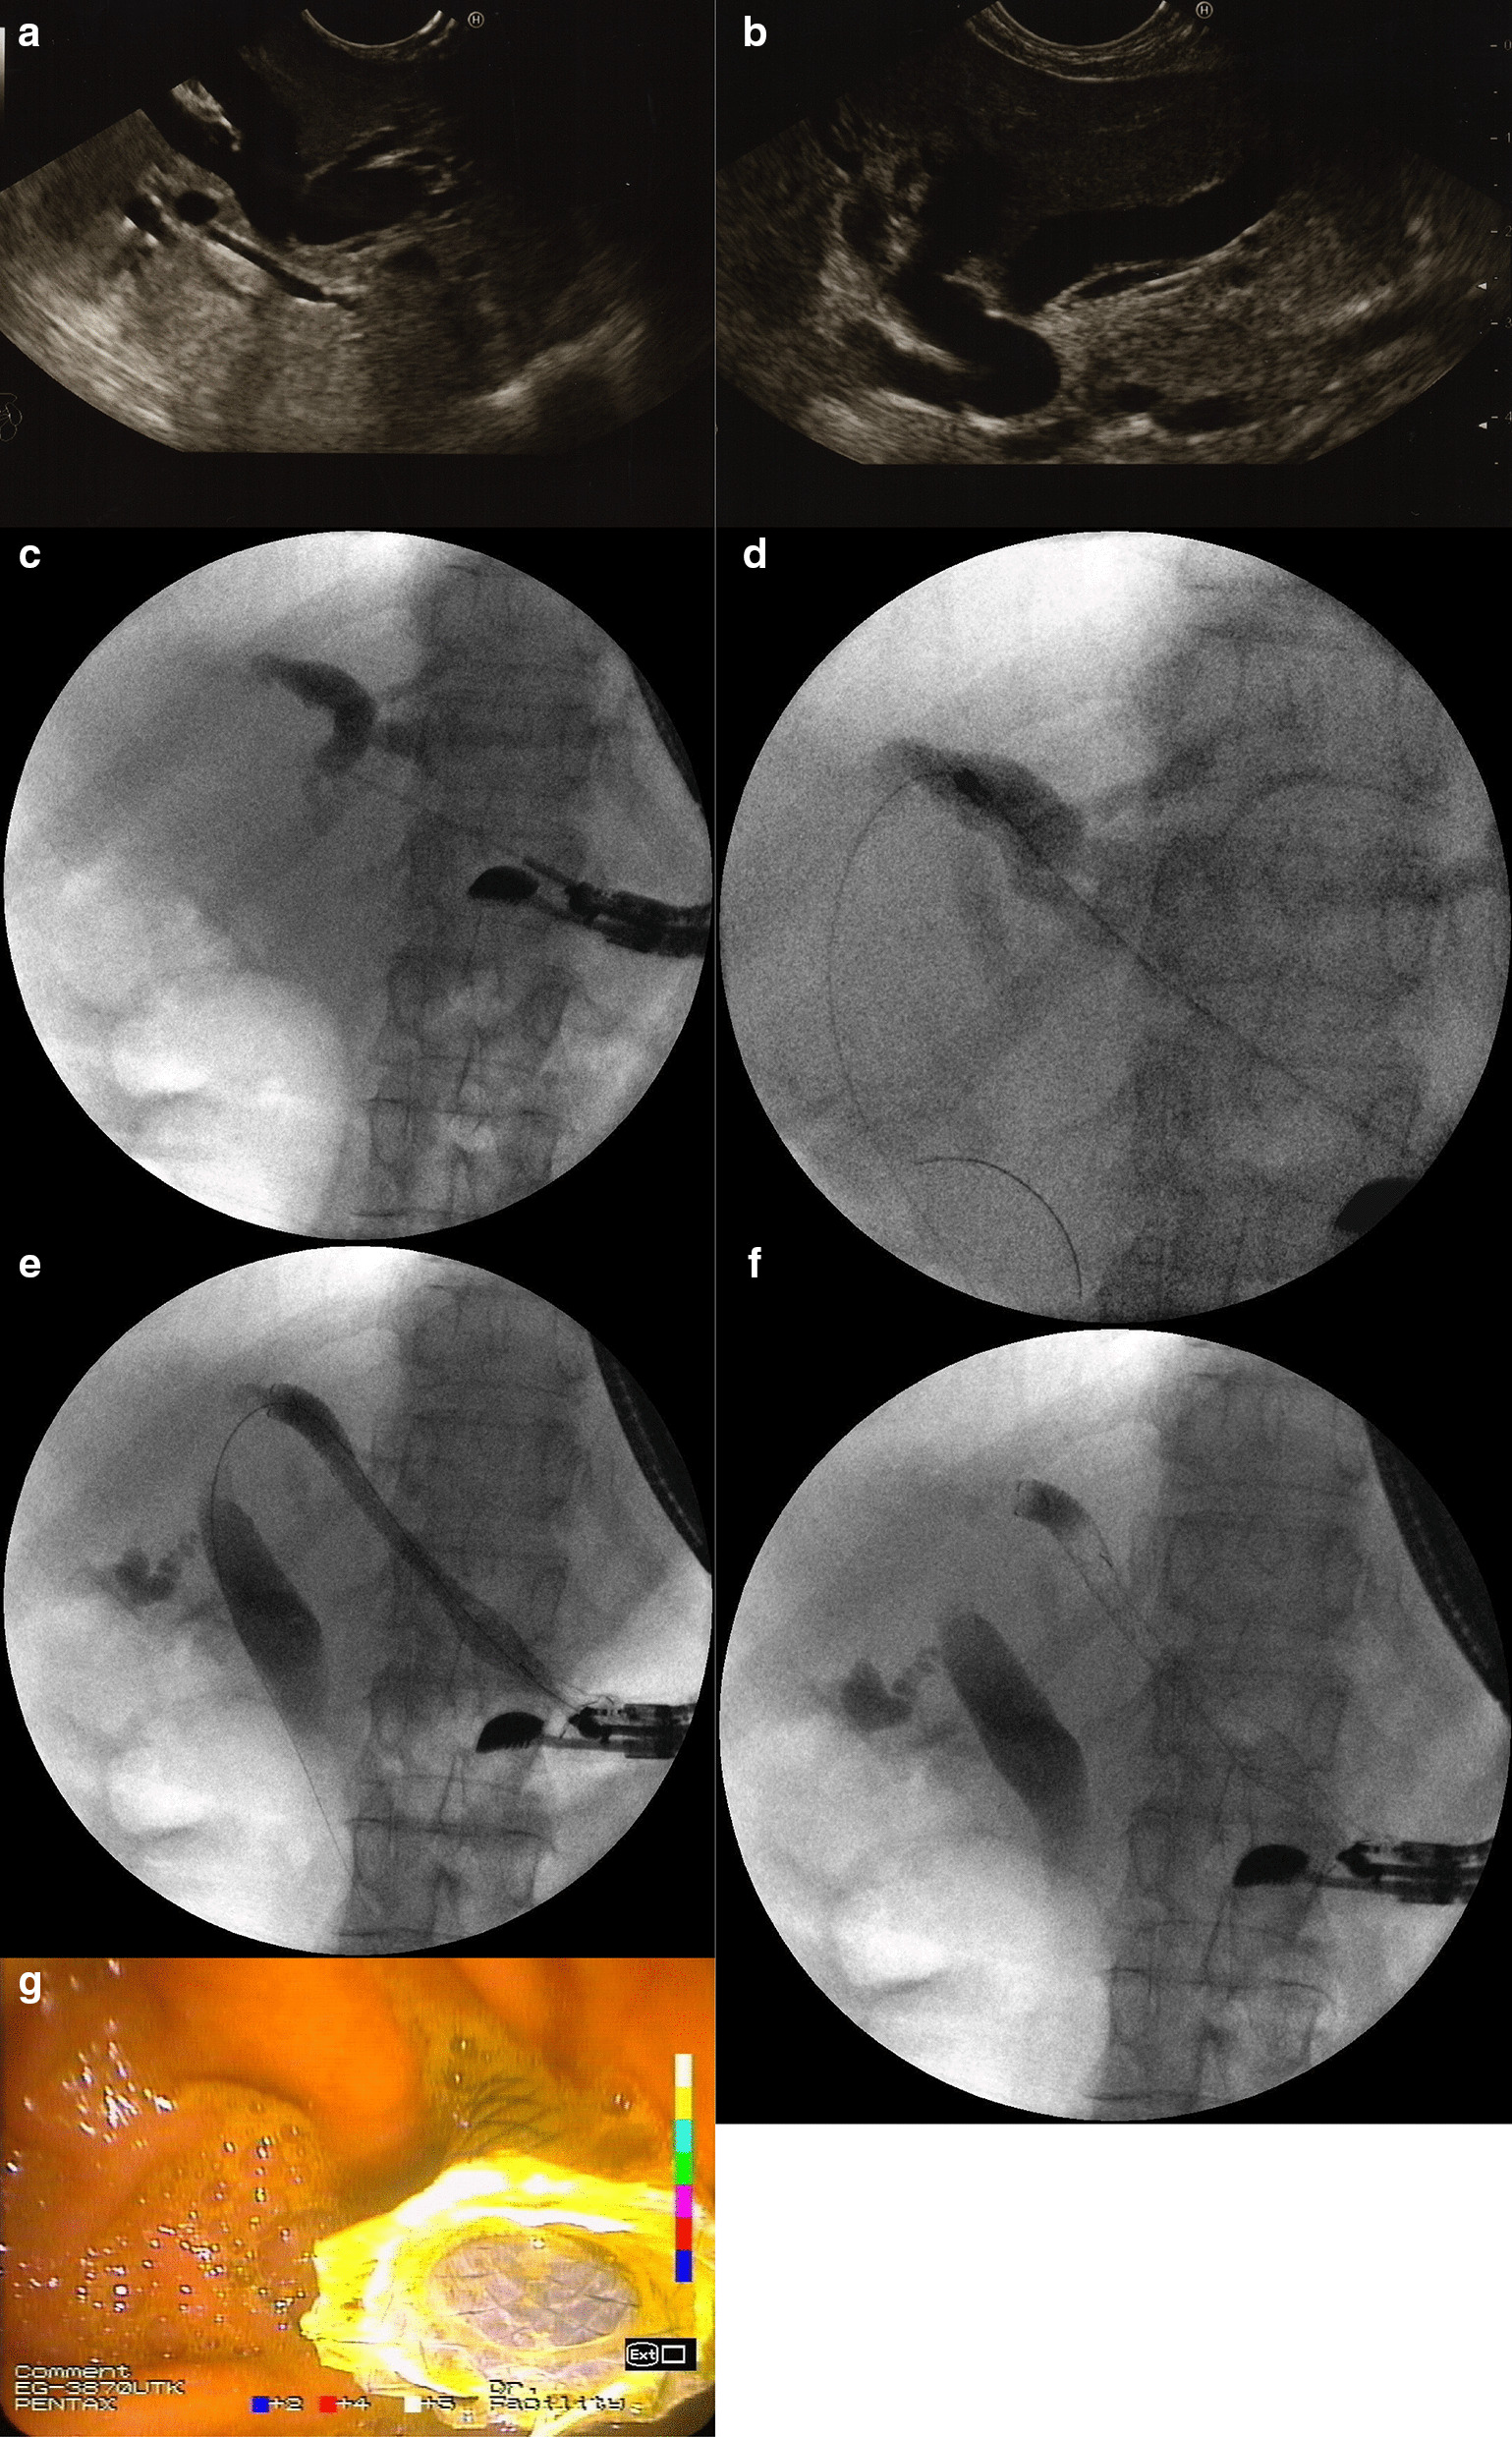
a–g EUS-guided hepaticogastrostomy in a patient with unresectable pancreatic head tumor (adenocarcinoma). Dilated bile ducts of left liver lobe visible in endosonographic image preceding endoscopic hepaticogastrostomy (a, b). Transmural puncture of the enlarged bile ducts within the left liver lobe was performed using a 19G needle and a contrast agent filled the enlarged bile ducts (c). A guidewire was introduced into the left bile duct and was directed towards the main bile duct. A 10 Fr cystostome was used to established a hepaticogastric fistula (d). Half-coated self-expandable endoprosthesis was introduced via the fistula (e–g)
